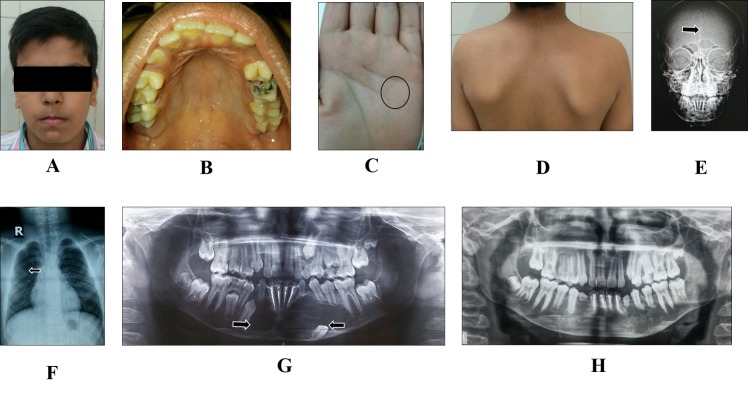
(A) Extraoral profile; (B) Intraoral examination; (C, D) Physical examination; (E) PA mandible radiograph; (F) Chest radiograph; (G) Panoramic radiograph; (H) Postoperative radiograph
